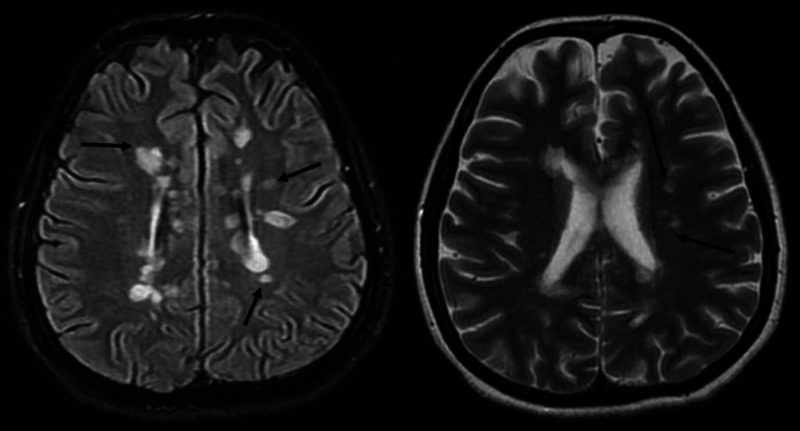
Axial FLAIR T-2 weighted images of the patient’s hemisphere, showing multiple hypointense and hyperintense periventricular lesions, typical of demyelination (black arrows). FLAIR: Fluid attenuated inversion recovery.
